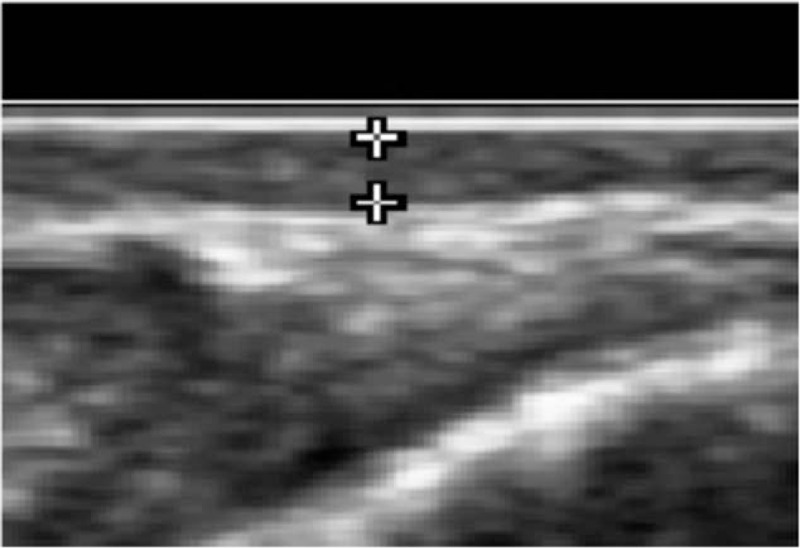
B-mode ultrasound measuring maximum scar thickness. Scar and subcutaneous tissue are visible in the image as a superficially limited hypoechoic layer and from the deep hyperechoic bands.
